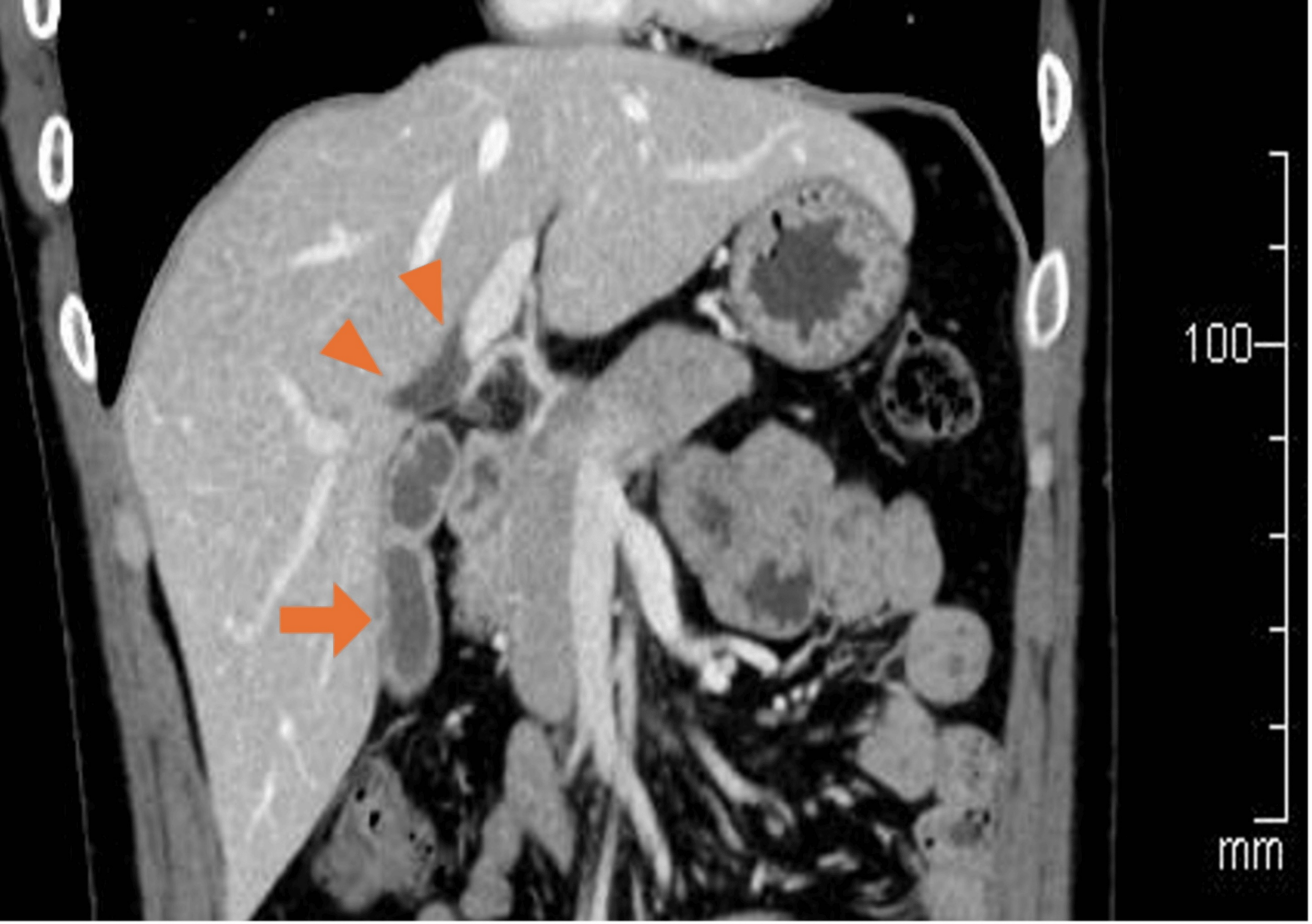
Computed tomography image showing a thickening of the gallbladder wall (arrow) and the dilated bile ducts in the hilar area (arrowheads)
